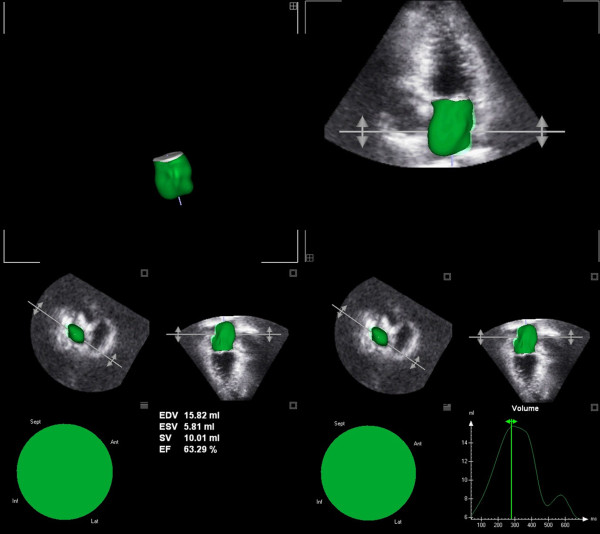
Three-dimensional echocardiographic LA reconstruction in a healthy subject: Normal LA volume and normal LAEF.
